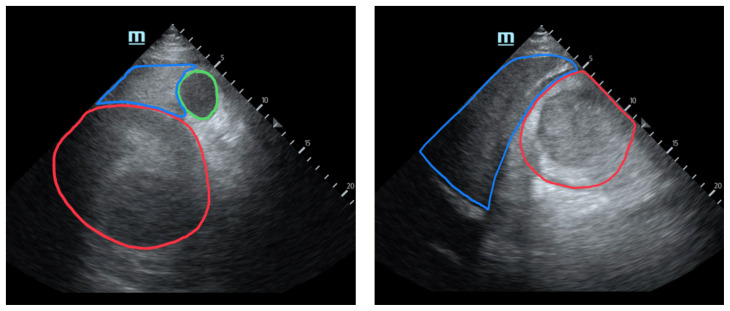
Unannotated Ultrasound 1 Video Link: https://youtu.be/PpEbnE6BDUo Annotated Ultrasound 1 Video Link: https://youtu.be/vj4mDGYWUH4 Unannotated Ultrasound 2 Video Link: https://youtu.be/mWY5-pl_9Do Annotated Ultrasound 2 Video Link: https://youtu.be/IWypm1ckbyg
